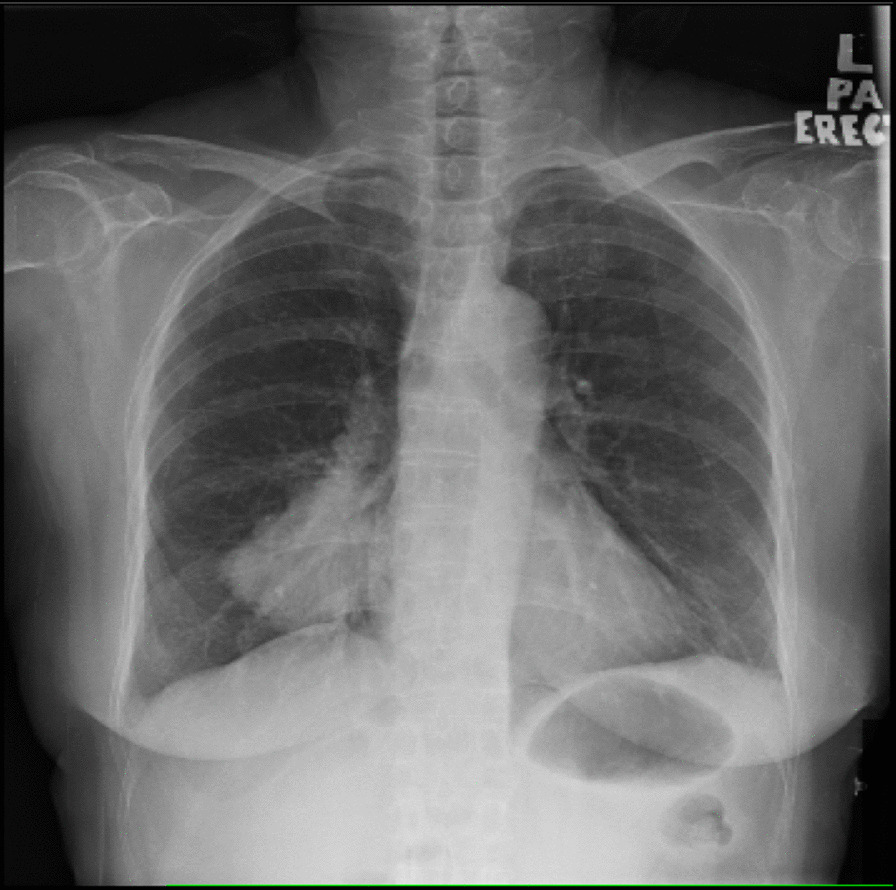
Chest X-ray (posteroanterior erect view) with mass over the right lower lobe
